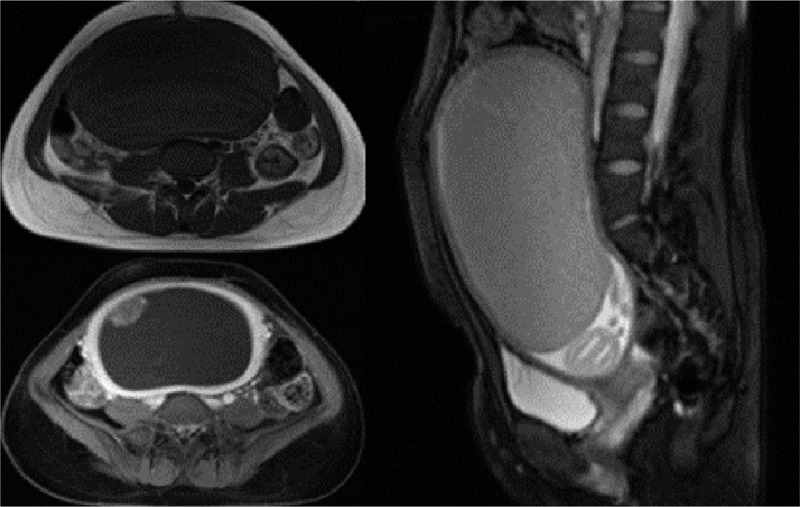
The pelvic MRI showed a 17.6cm × 8.4cm × 16.9 cm mass with long T1 and slightly long T2 signals in the uterus, of which only a small part was enhanced under contrast, and the boundary around the mass separated it clearly from the uterus wall. Still could the fetus be found at the internalorifice of cervix. MRI = magnetic resonance imaging.
